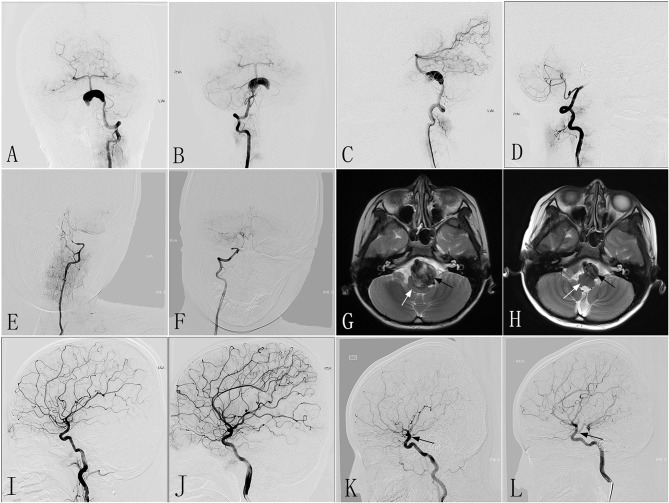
Images from an 8- to 10-year-old patient with a giant dissecting aneurysm located in the VBJ (case 3). (A,B) Preoperative anteroposterior angiograms of the LVA (A) and RVA (B) showing a giant dissecting aneurysm in the VBJ. (C,D) Immediately postoperative lateral angiograms showing the successful reconstruction of the LVA (C) and the complete occlusion of the RVA by coiling (D). (E,F) Six-month post-treatment angiograms confirming the complete occlusion of the LVA (E) and the RVA (F). (G,H) Six-month post-treatment MRI (H) compared with MRI performed 1 day post-treatment (G) demonstrating the reduction in the aneurysm size (black arrow) and increased space around the brainstem (white arrow). (I,J) Preoperative angiograms of the left and right ICA demonstrating weak dual-sided PCoAs. (K,L) Six-month post-treatment angiograms of the left and right ICA confirming robust dual-sided PCoAs (black arrow) providing sufficient blood for posterior circulation. VBJ, vertebrobasilar junction; LVA, left vertebral artery; RVA, right vertebral artery; ICA, internal carotid artery; PCoAs, posterior communicating arteries.
